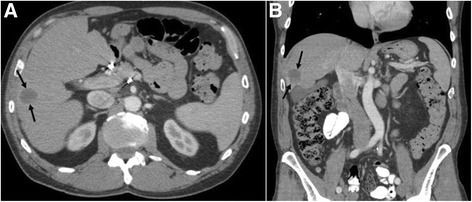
a Axial and b coronal post-contrast CT images through the abdomen during PD-1 therapy again demonstrate the index metastatic lesion (*arrows*) within the right hepatic lobe with post-treatment changes and marked decrease in size
